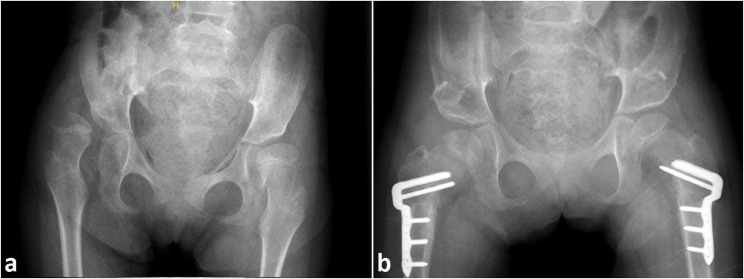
Pelvic radiographs. (a) showing the preoperative condition: dislocated right and subluxated left hip; (b) postoperative outcome at the 2-year follow up: VDRO and Dega osteotomy on both sides with reduced and stable hips
